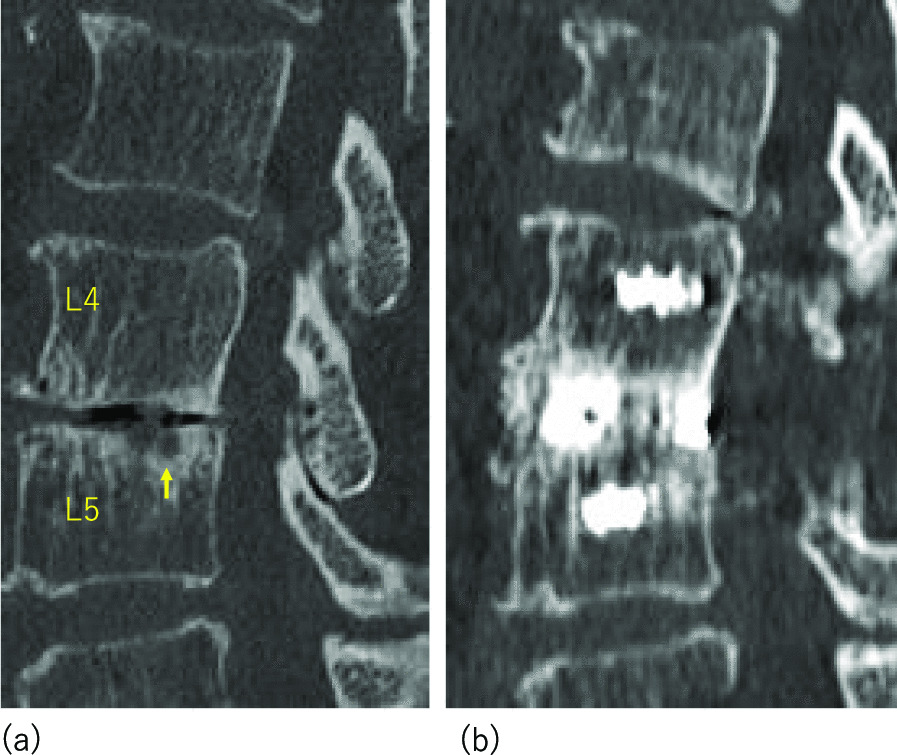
Representative case of an 81-year-old woman with stenosis of the lumbar spinal canal. a Preoperative sagittal lumbar computed tomography (CT) shows vertebral endplate cyst formation (VECF) (↑arrow) in the upper endplate of the L5 vertebra. b Sagittal lumbar CT performed 6 months after L4/5 posterior fusion shows intervertebral bony fusion, no halation around the cage, and complete VECF resolution
